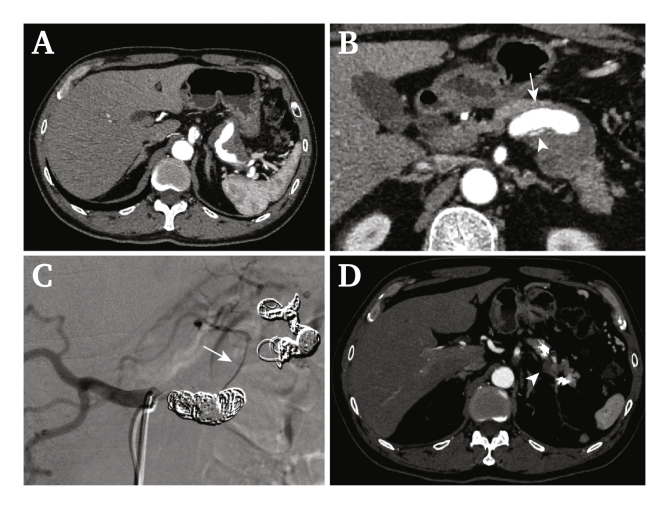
(A) Computed tomography (CT). Coeliac artery dissection spread to the splenic artery and formed a splenic aneurysm. (B) The false lumen (arrow) severely oppressed the true lumen (arrow head) but re-entered it, causing no ischaemia. (C) Angiography. The false lumen was successfully embolised using the isolation technique, and the true lumen (arrow) was preserved. (D) Follow up CT. The false lumen was successfully thrombosed to shrink (arrow head) and splenic infarction was limited to a small area.
